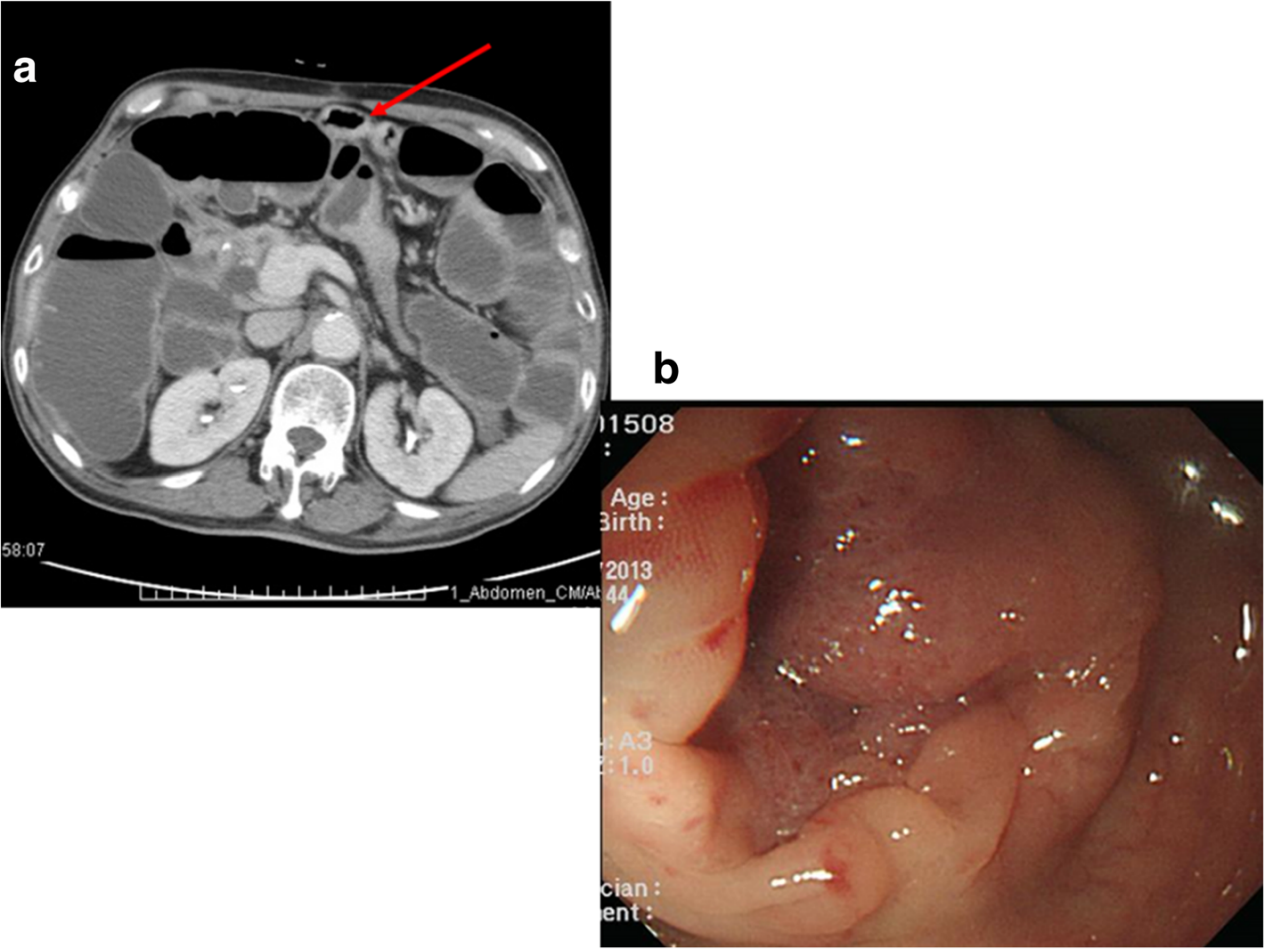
Metachronous colonic metastasis of advanced GC. a Abdominal CT showed a circumferential mass lesion with severe distention of the proximal colonic loop and small bowel loop, suggesting colonic metastasis. b Colonoscopy revealed mucosal swelling with total obstruction
